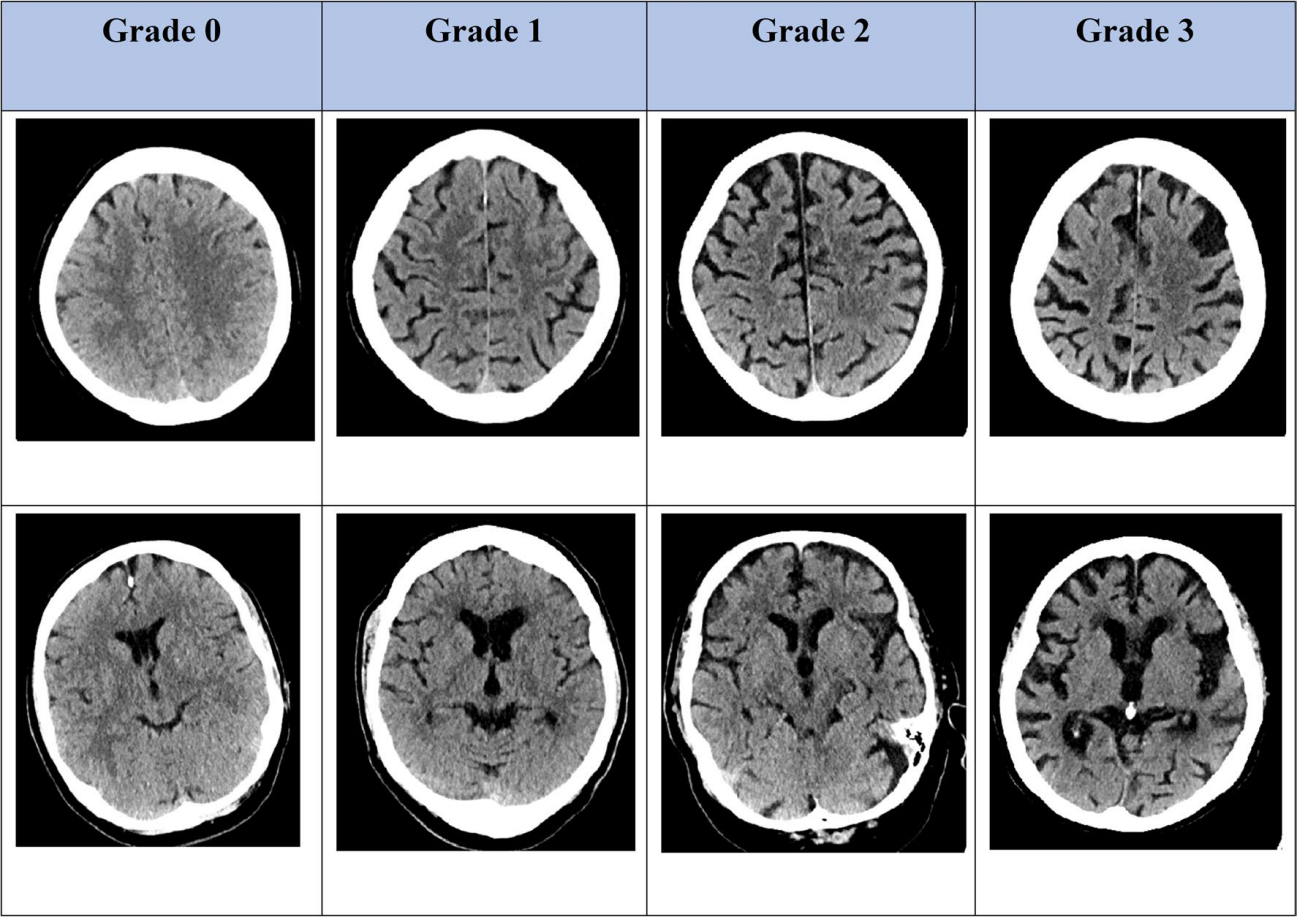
Visual examples for atrophy grading on CT images. Grade 1—minor atrophy to the insular and/or the frontoparietal region, cerebral sulci slightly more pronounced. Grade 2—moderate atrophy, further widening of the cerebral sulci. Grade 3—global atrophy, severely widened sulci, thinning of the cortical gyri. Windowing was set at L40/W60 for all images
